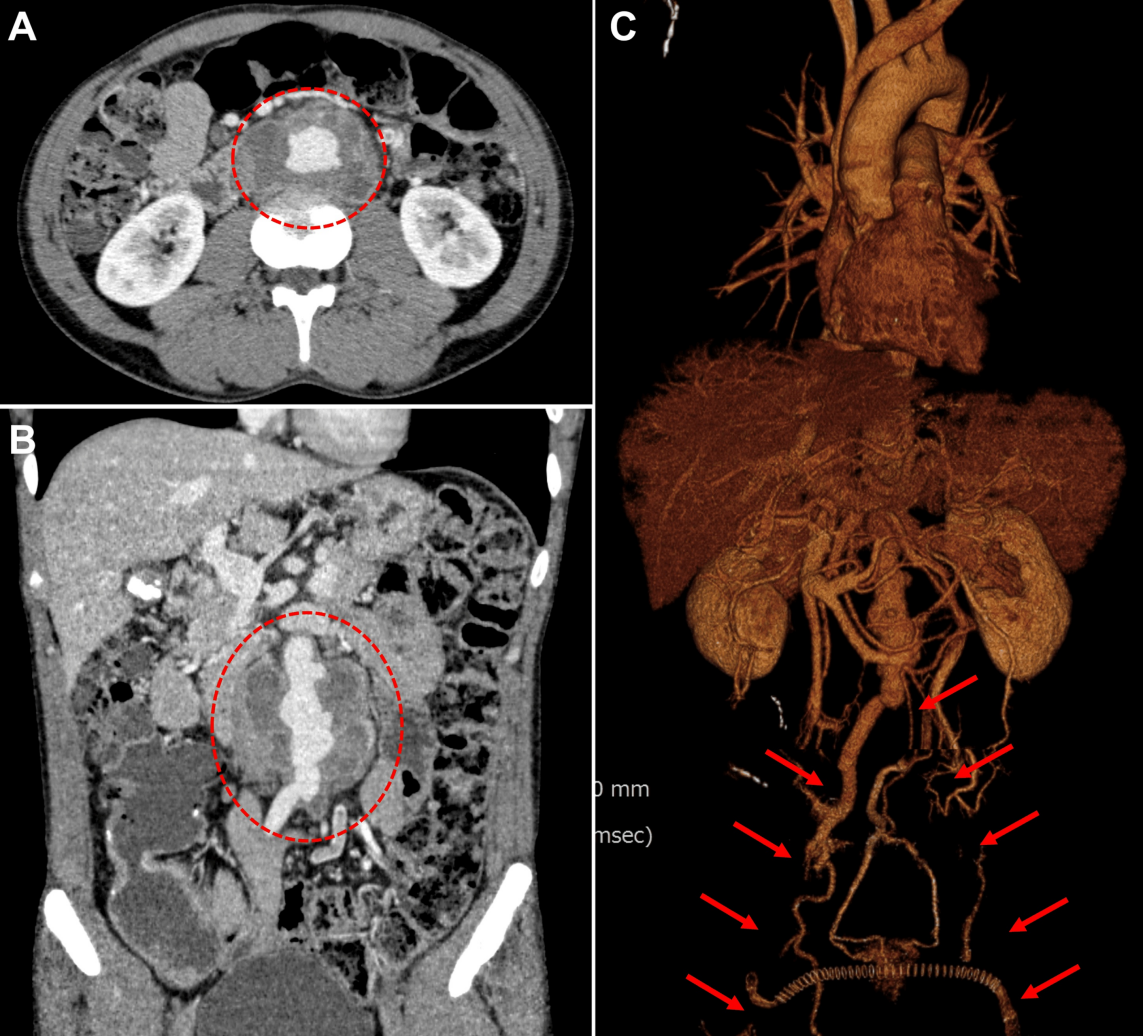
Preoperative computed tomography images (A) Axial, (B) coronal, and (C) three-dimensional images showing an irregularly shaped AAA (circles) and occluded bilateral lower extremity arteries (arrows). AAA: abdominal aortic aneurysm
